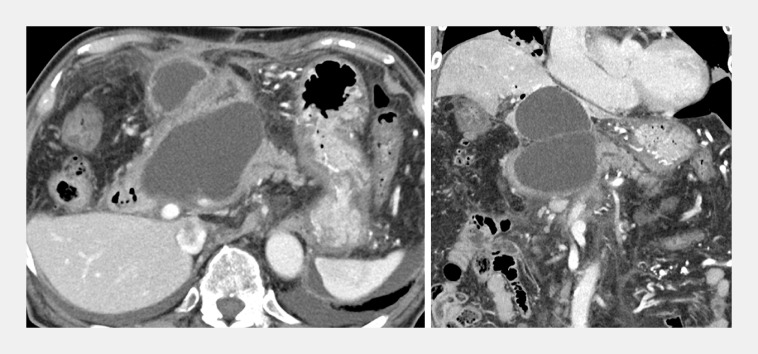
Computed tomography (CT) showing a large pancreatic fluid collection at the pancreaticojejunal anastomosis site following modified Child resection in a 70-year-old man.
